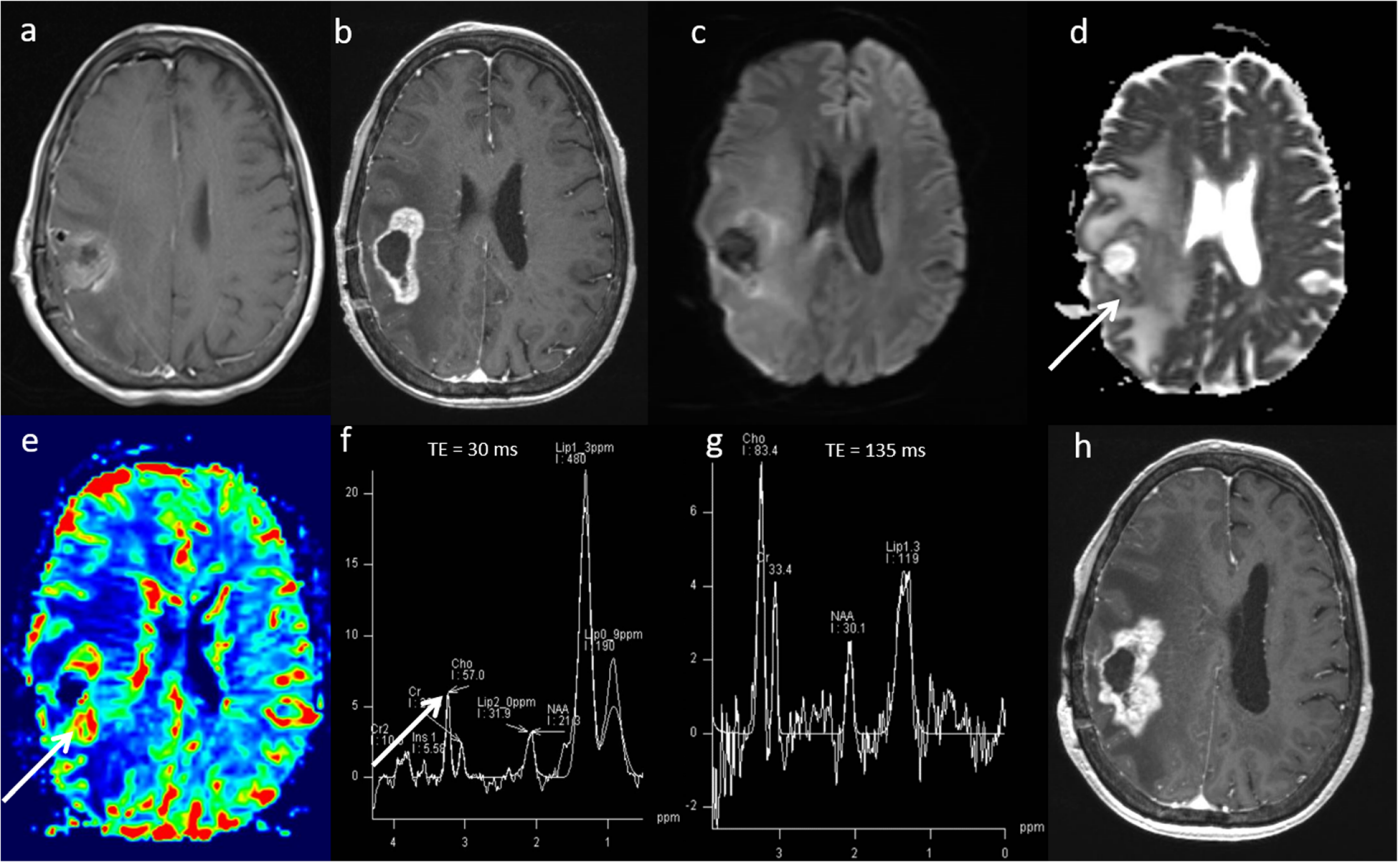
Glioblastoma—true progression. a Immediate post-operative contrast-enhanced T1-weighted scan following resection of a right fronto-parietal glioblastoma. b Conventional post-contrast T1-weighted image one month after chemoradiotherapy treatment demonstrates increase in the enhancing lesion size with associated oedema. Multiparametric MRI at this timepoint demonstrates: c, d areas of low ADC (903 × 10^−6^ mm^2^ s^−1^, arrow), (e) a high rCBV ratio (3.0, arrow) on PWI, (f, g) a high Cho/Cr ratio (2.3, arrow), high Cho/NAA ratio and presence of lipid/lactate on MRS. All parameters suggest a poor response and disease progression. h Six-month follow-up conventional post-contrast T1W sequence confirms an increase in enhancing disease, indicating true progression
